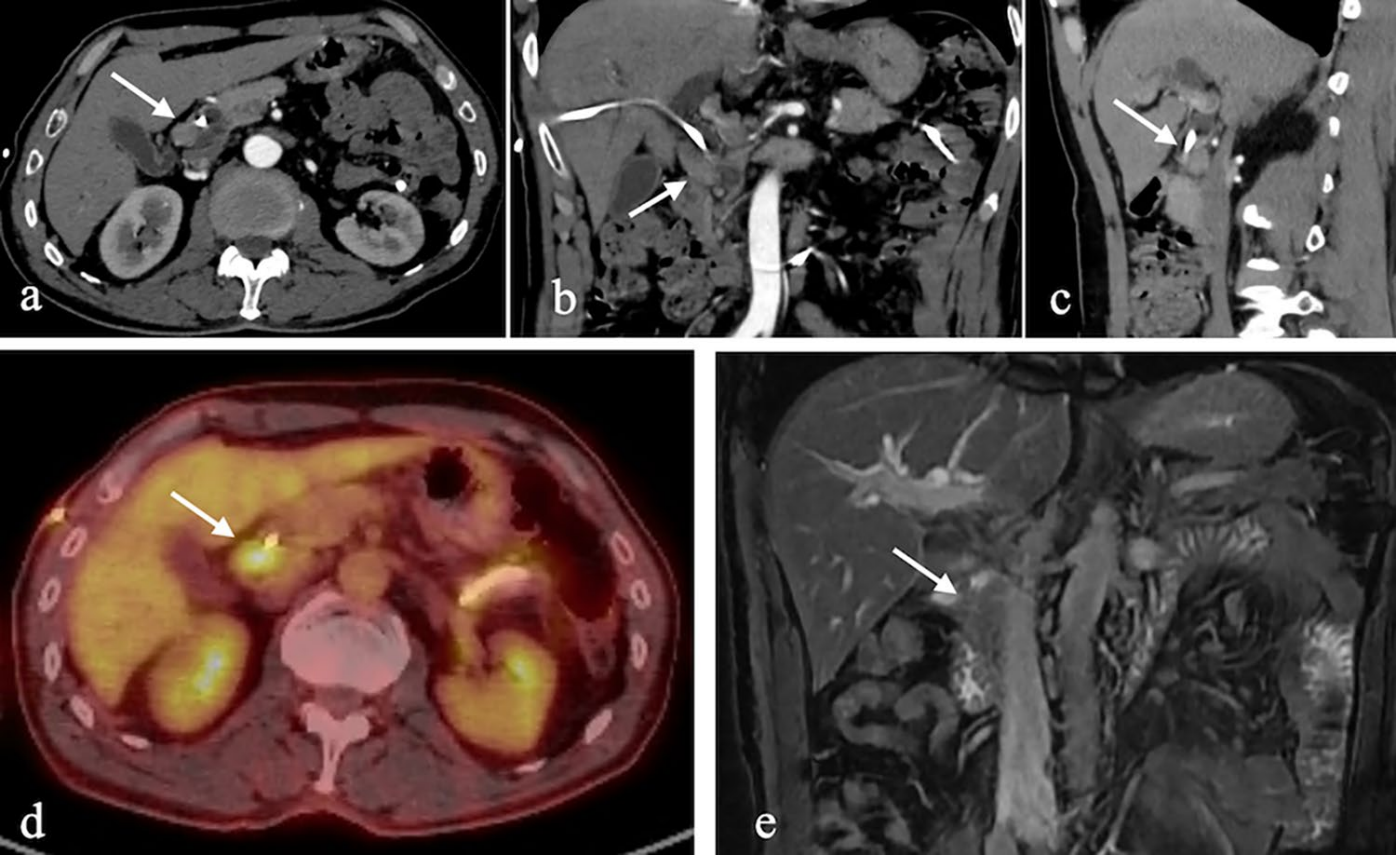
a. Axial, b. Coronal and c. Sagittal IV contrast-enhanced arterial-phase CT images of a 63-year-old male patient, showing a hypervascular nodular lesion in the second (D2) segment near the major papilla. d. FDG-PET/CT fusion image showing high FDG uptake. e. Coronal T2-weighted MRI image demonstrating hypointensity of the lesion. Diagnosis: neuroendocrine tumor
